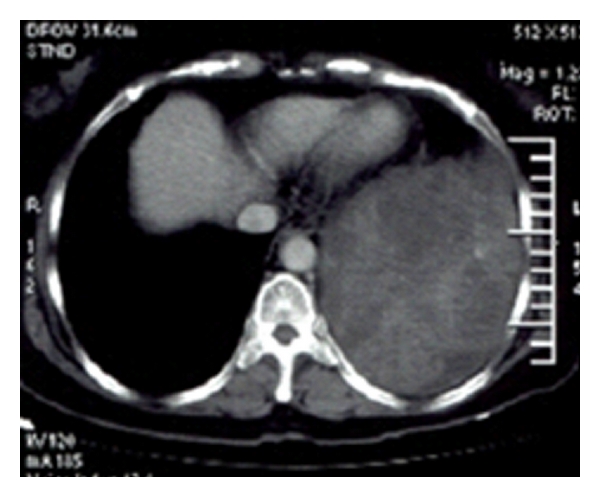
CT findings of angiosarcoma of the diaphragm (tumors were located under the chest, a huge volume, heterogeneous enhancement, No mediastinal lymph nodes).
